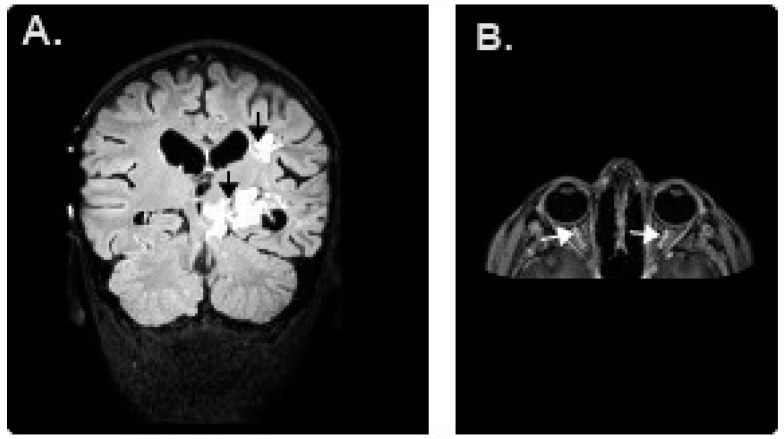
(A) Magnetic resonance image (MRI) of the patient’s brain demonstrating a left brain hemisphere and brainstem glioblastoma; (B) On T1-weighted sequence, after contrast injection, an enhancement of the bilateral optic nerve compatible with optic neuritis.
